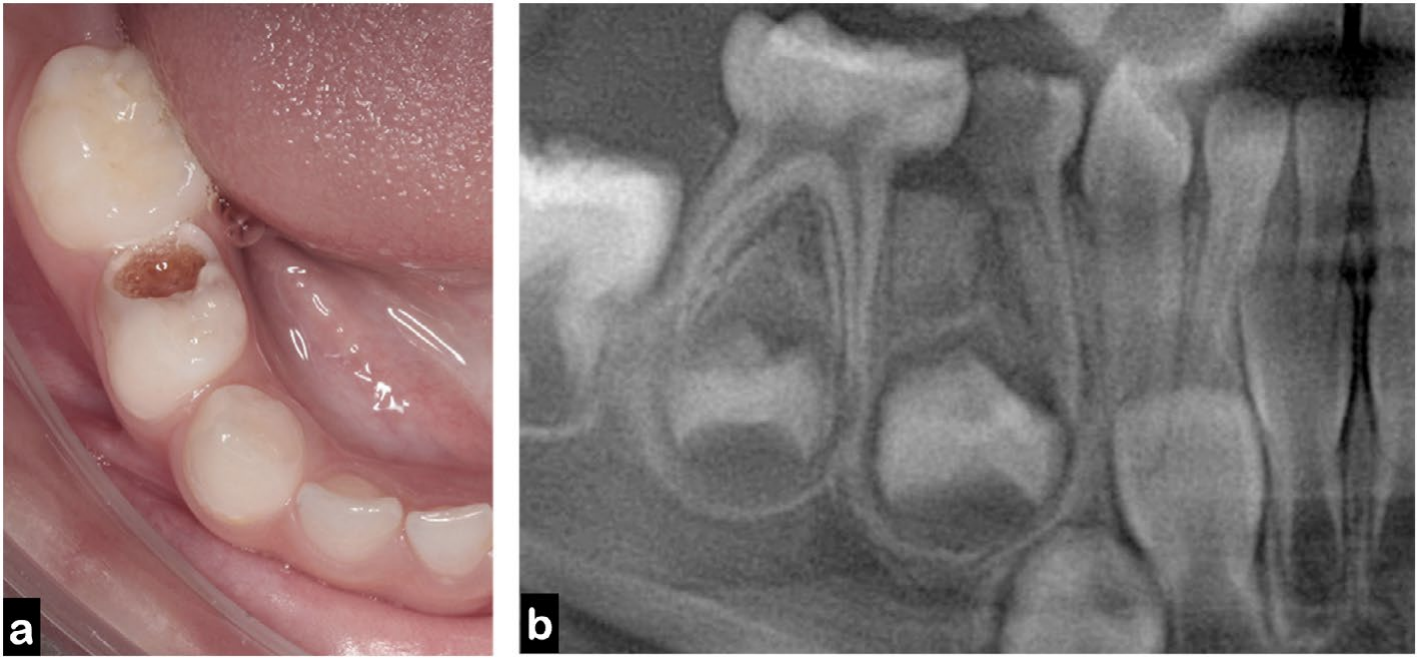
Initial clinical and radiographic findings: (a) intraoral lateral view showing extensive carious destruction of tooth 84 with furcation involvement; (b) periapical radiograph confirming irregular distal root resorption and early developmental stage of the permanent successor (tooth 44), classified as Nolla stage 5.
